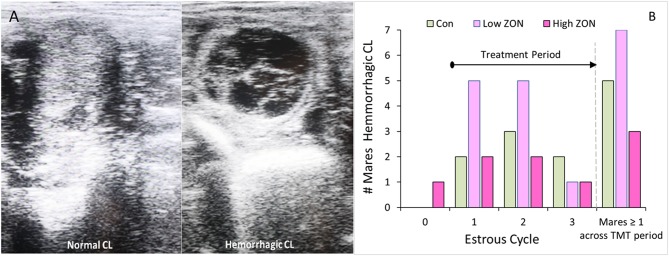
Corpus luteum development: (A) Ultrasound comparison of Normal and Hemorrhagic CL; (B) Incidence of hemorrhagic corpora lutea (HCL) by cycle, such that time on ZON treatment increases with cycle number. The last set of bars: number of mares by treatment having at least one HCL over entire study period. ZON treatments are: Control (*n* = 6) 0 mg/da, Low (*n* = 7) 2 mg/da, High (*n* = 7) 8 mg/da.
